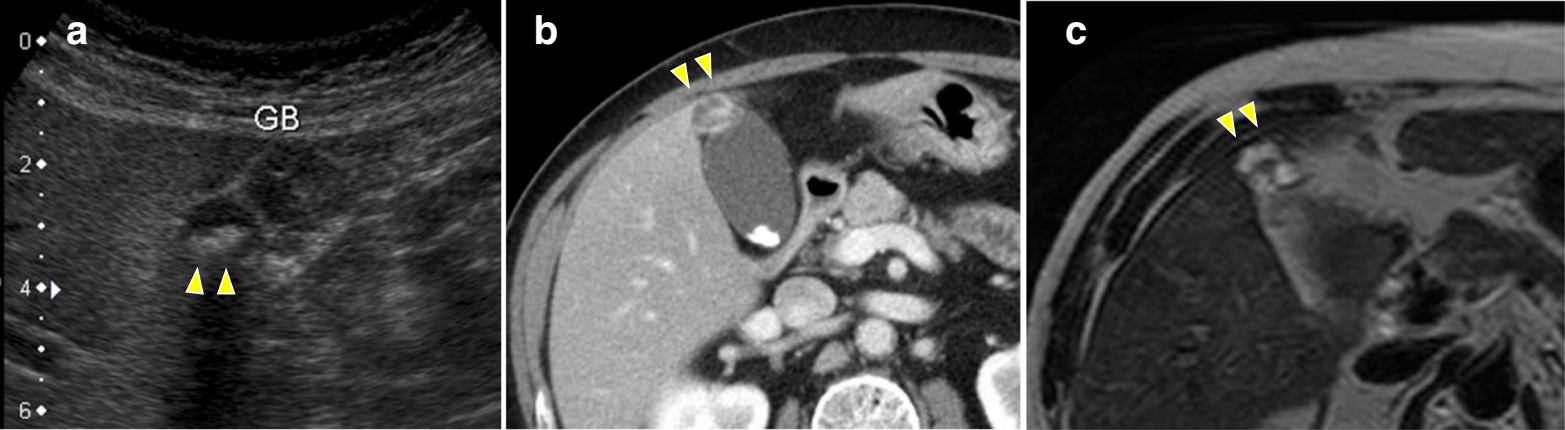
Preoperative imaging findings. a Abdominal ultrasonography showed an 8 × 7-mm solid mass at the gallbladder fundus. b Enhanced CT showed that irregular wall thickening at the gallbladder fundus, and the boundary between the tumor and liver was indistinct. c T2-weighted MRI showed high-intensity nodules inside the thickened wall of the gallbladder
